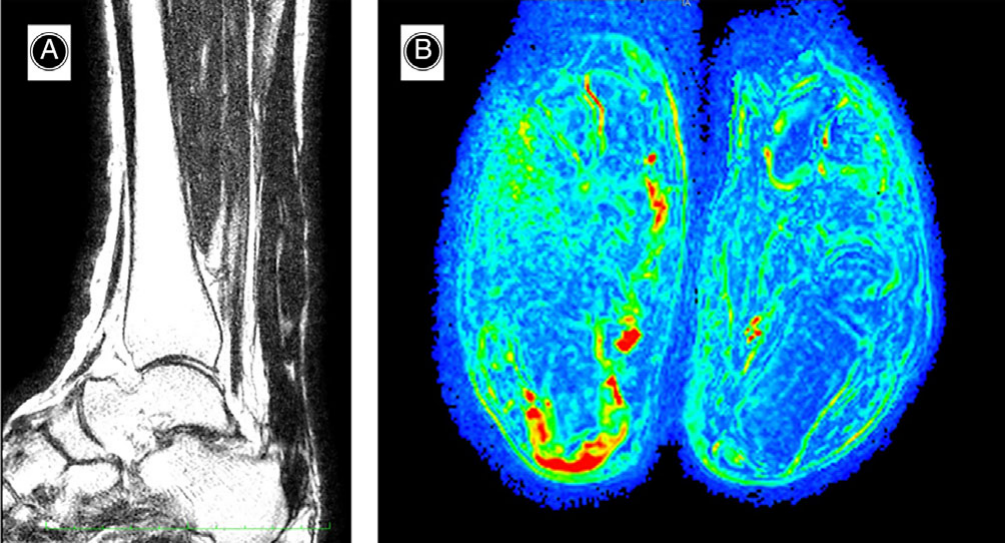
(A) Conventional sagittal MRI study with altered Achilles tendon signal. (B) Dynamic perfusion MRI study. Signs of incomplete tendon healing are evident in the left image. Right image healthy side.
